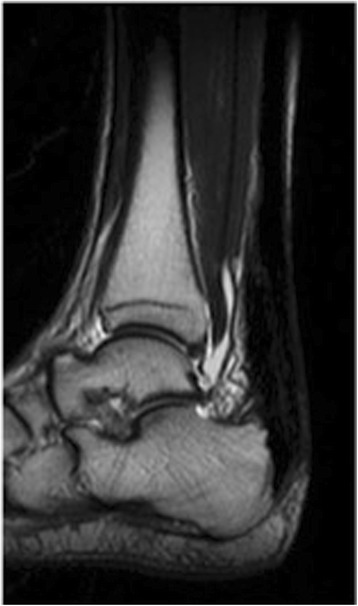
Magnetic resonance imaging scan obtained at 6 postoperative months. Continuity of the Achilles tendon was observed
